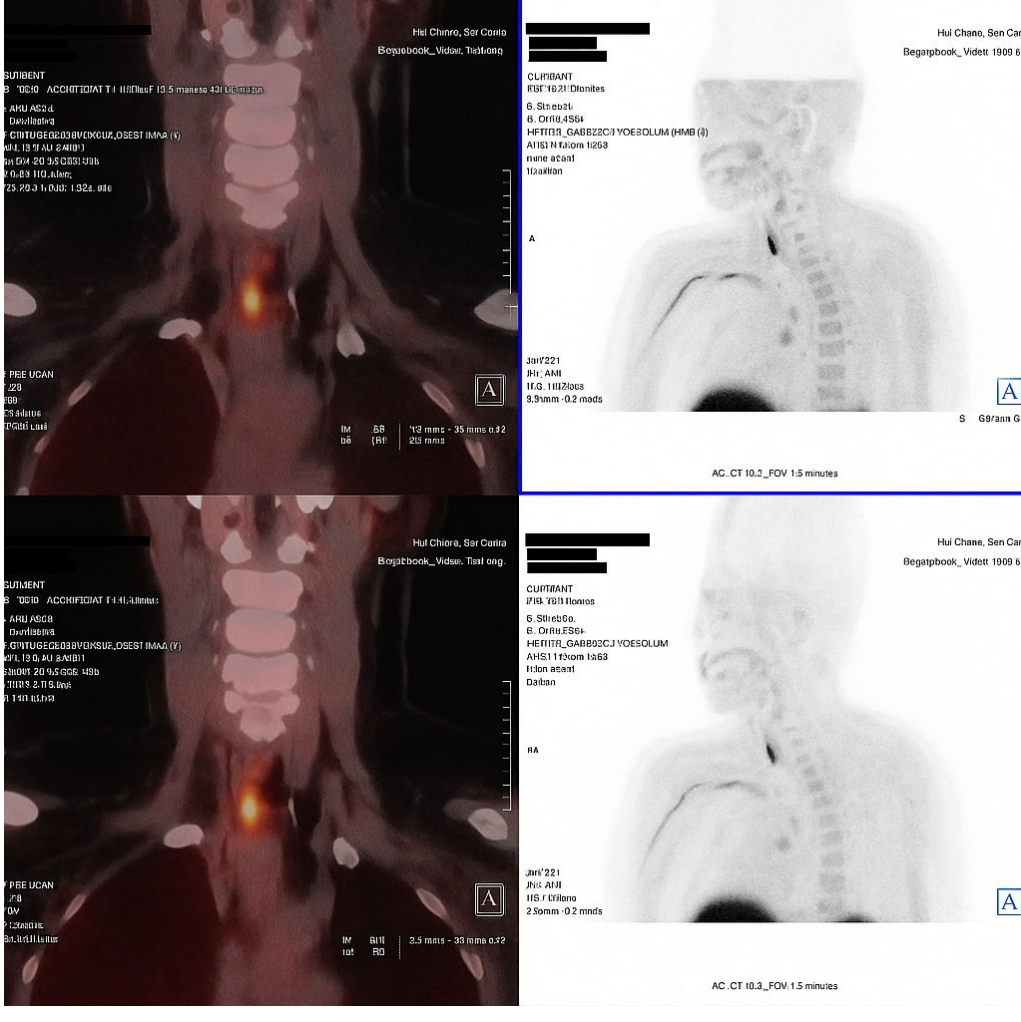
Fused coronal and axial images (left) and anterior projection MIP sections (right) identify two areas of focal increased uptake in the right cervical region. The uptake corresponds to a posterosuperior nodular lesion and an inferoposterior nodular lesion adjacent to the right thyroid lobe, findings consistent with double right parathyroid adenomas (superior and inferior). This technique localized the parathyroid adenomas, eliminating the need for FDG-PET to exclude oncogenic osteomalacia.
